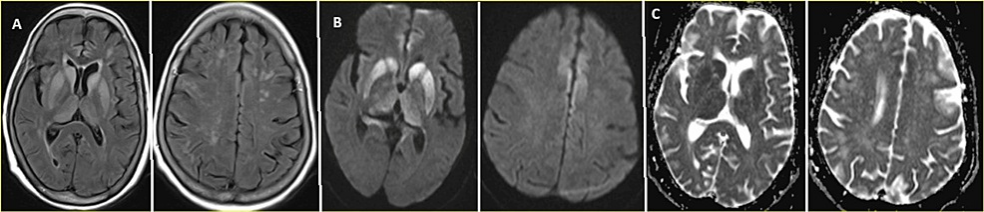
Magnetic resonance brain images of a patient with Creutzfeldt-Jakob disease: changes in deep gray matter and bilateral parasagittal regions MRI BRAIN FLAIR, DWI, and ADC axial sequences of patient 4 are shown. FLAIR sequence (first two images on the right (A)) shows hyperintensities in the bilateral caudate, putamen, and thalamus with diffusion restriction on DWI&ADC mapping (corresponding DWI sequences in the two images in the middle (B), corresponding ADC in the two images on the left (C)). Similar FLAIR hyperintensities with diffusion restriction were also noticed in bilateral cingulate gyrus Cortical diffusion restriction has cortical ribboning pattern. FLAIR hyperintensities with no diffusion restriction notes in subcortical and deep white matter of frontal and parietal lobes suggestive of probable small vessel/microvascular ischemic changes. MRI, magnetic resonance imaging; FLAIR, fluid-attenuated inversion recovery; DWI, diffusion-weighted imaging; ADC, apparent diffusion coefficient.
